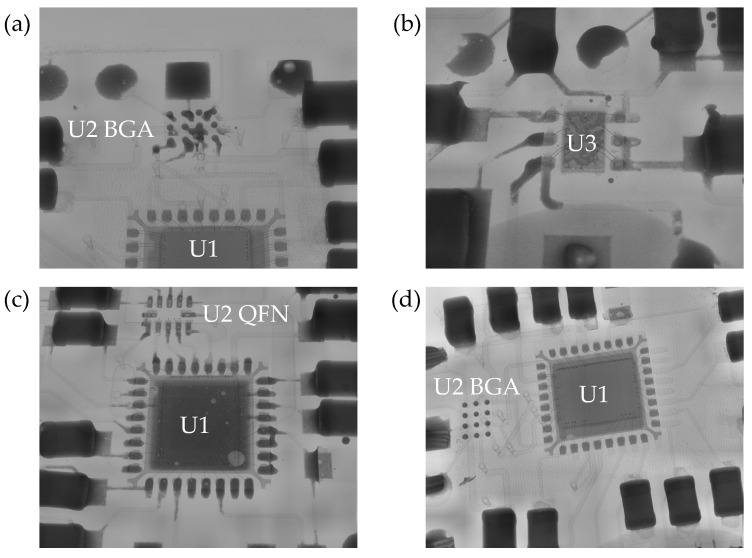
(a–d) X-ray scan of the resin circuit board.
